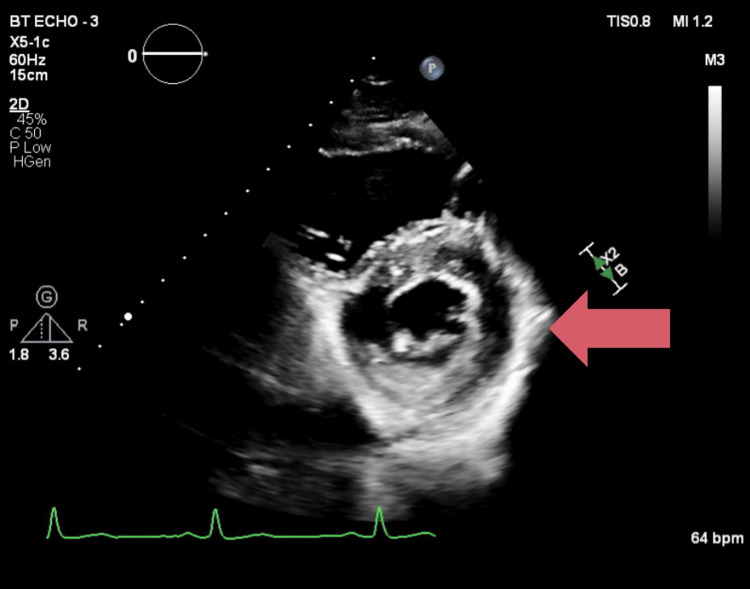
Transthoracic echocardiogram parasternal short axis view showing left ventricular concentric hypertrophy
